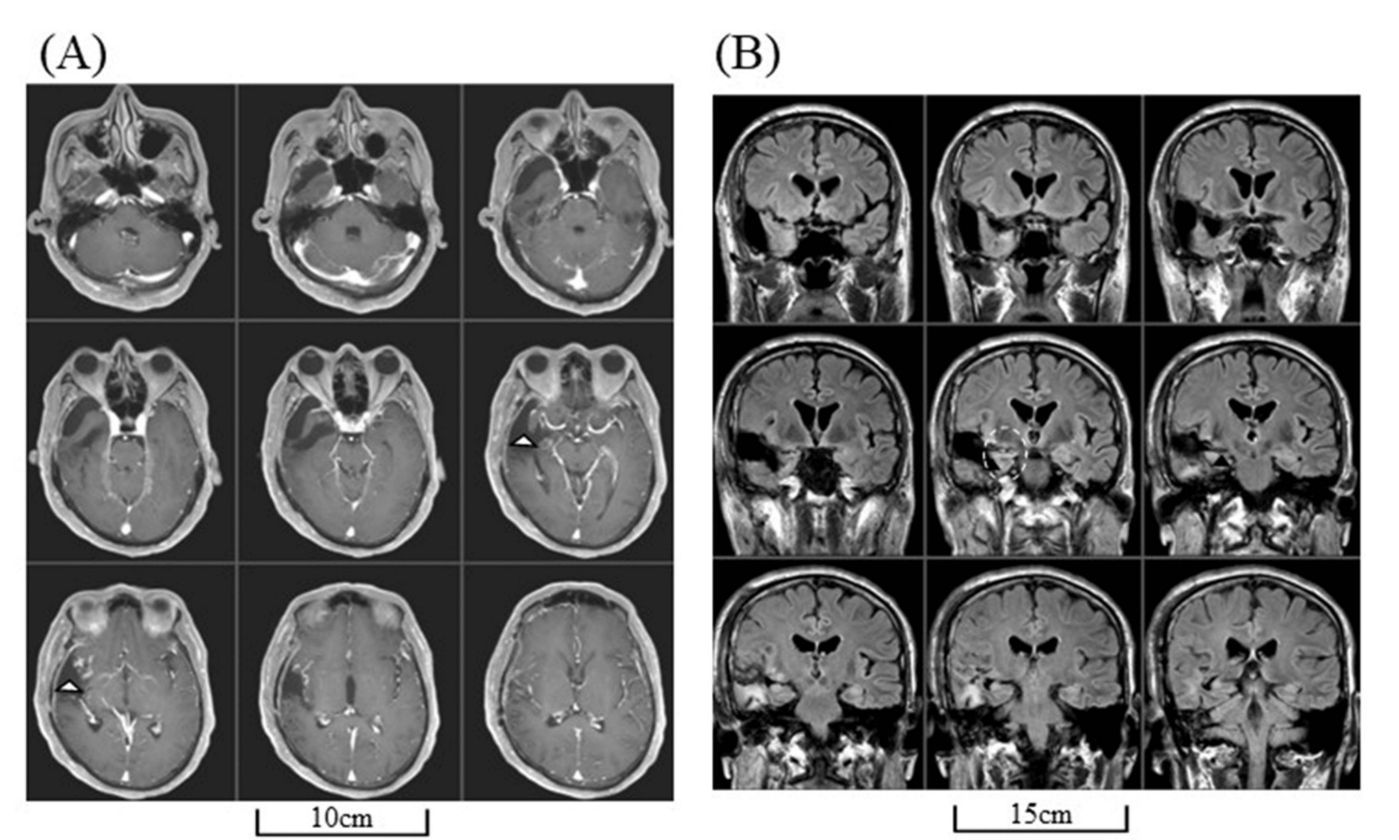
MRIs after initial treatment Head MRIs after initial treatments (i.e., surgery, chemotherapy with temozolomide, and radiation therapy) revealed no residual tumor in post-enhanced T1-weighted images (arrowheads) (A) and slight edema in the posterior part of the temporal lobe in FLAIR images (dotted circle) (B). FLAIR: fluid-attenuated inversion recovery; MRI: magnetic resonance imaging
